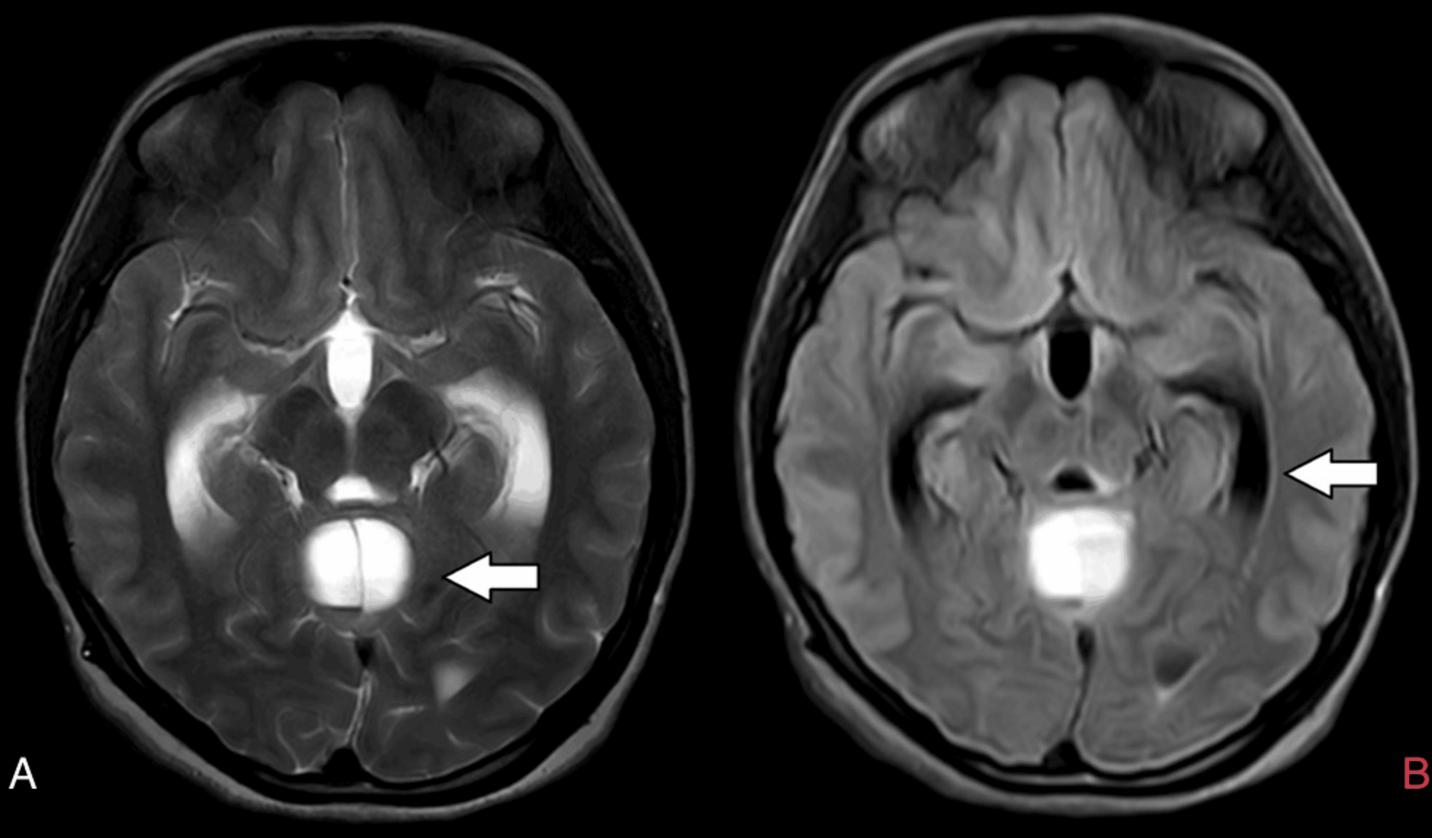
Axial T2 and FLAIR MRI brain images A) Arrow shows dilatation of the fourth ventricle; B) Arrow shows dilatation of the temporal horn of the lateral ventricle. FLAIR: Fluid-attenuated inversion recovery; MRI: Magnetic resonance imaging
